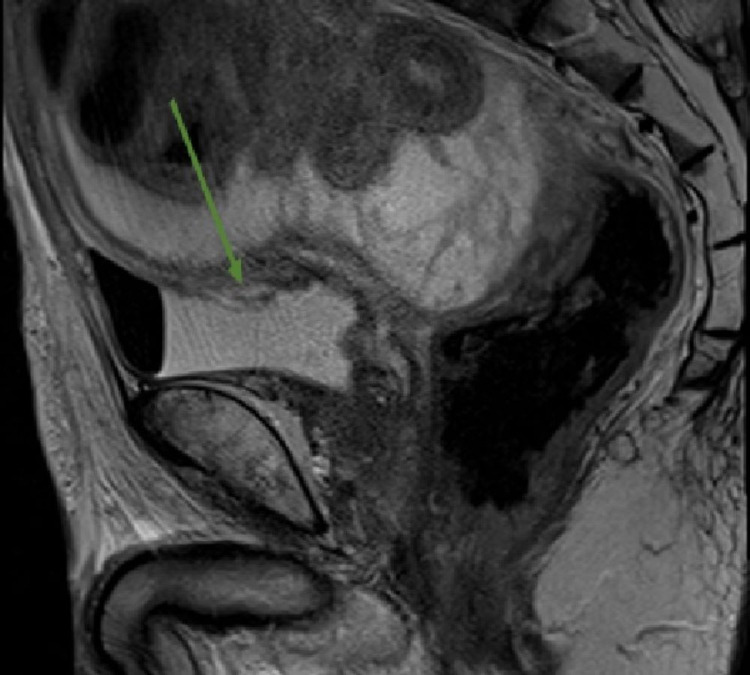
Pelvic magnetic resonance imaging on day four Pelvic T2-weighted magnetic resonance imaging revealed high signal intensity in the dome of the bladder, indicating rupture of the bladder wall (arrow).
